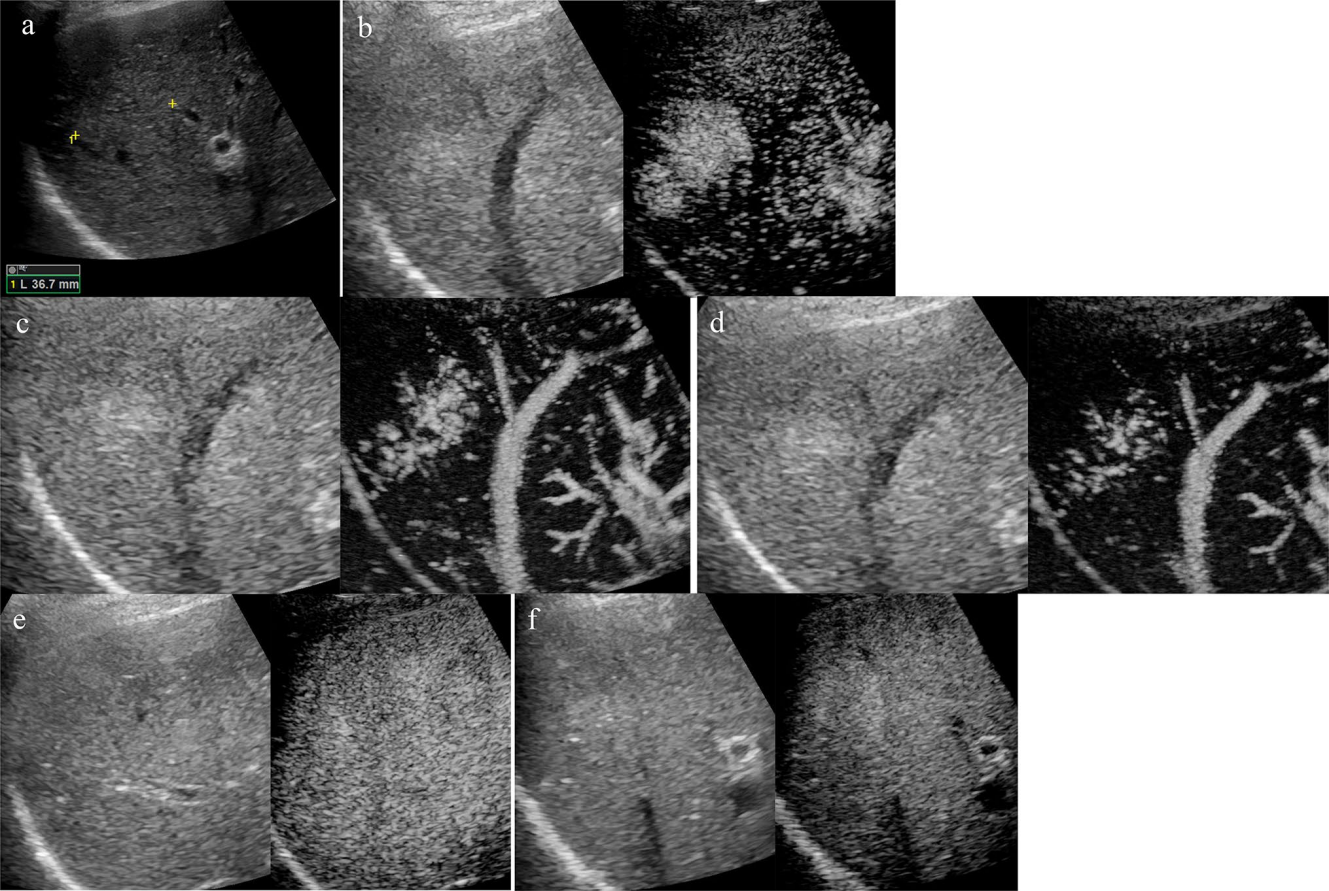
Ultrasound examination. a B-mode ultrasound showed a 35-mm mass lesion as a slightly hyperechoic area with unclear borders in segments 6 and 7. b Contrast-enhanced ultrasound showed that the entire lesion was enhanced in the arterial phase. c, d Perfusion images showed that the enhancement pattern of fast centrifugal filling of the lesion was composed of central vessels and radical vascular branches (spoke-wheel appearance). e In the post-vascular phase, there was no defect in the lesion. f The mass became iso-enhanced in the portal phase
